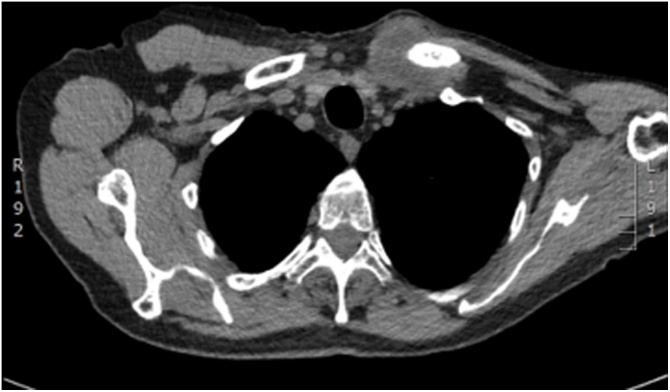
MRI of the upper thorax and brachial plexus. A cystic mass with infilterating nodular enhancing walls centred at head of clavicule, infiltrates the clavicular and sternal heads of the left sternocleidomastoid muscle, the sternohyoid muscle inferiorly and the medial end of the first rib. The clavicle is infiltrated from the head medially to the mid-third laterally over a distance of at least 6 cm. In the neck and posteriorly the left ICA is well clear of the mass, but the distal left IJV is not well seen. Subclavian vein and distal vertebral vein run immediately posterior to the mass and are inseparable from it. Subclavian artery and brachial plexus do not appear to infiltrated.
